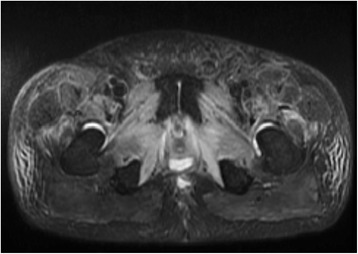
MRI pelvis post-radiation, axial STIR/T2W FSE sequence showing edema of proximal leg muscles
